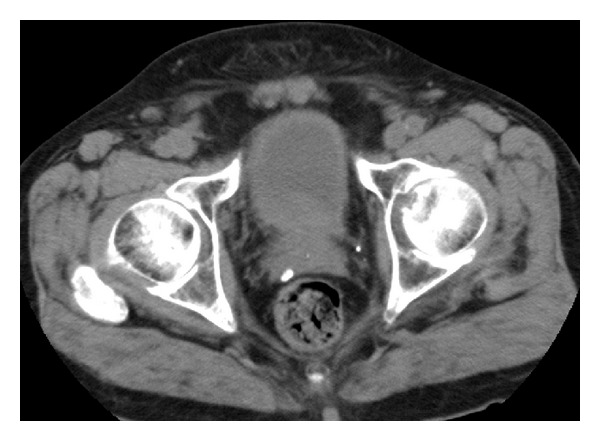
Precontrast CT demonstrated a diminution of the size of the lymphoma of the prostate 25 months after the prostate biopsy.
